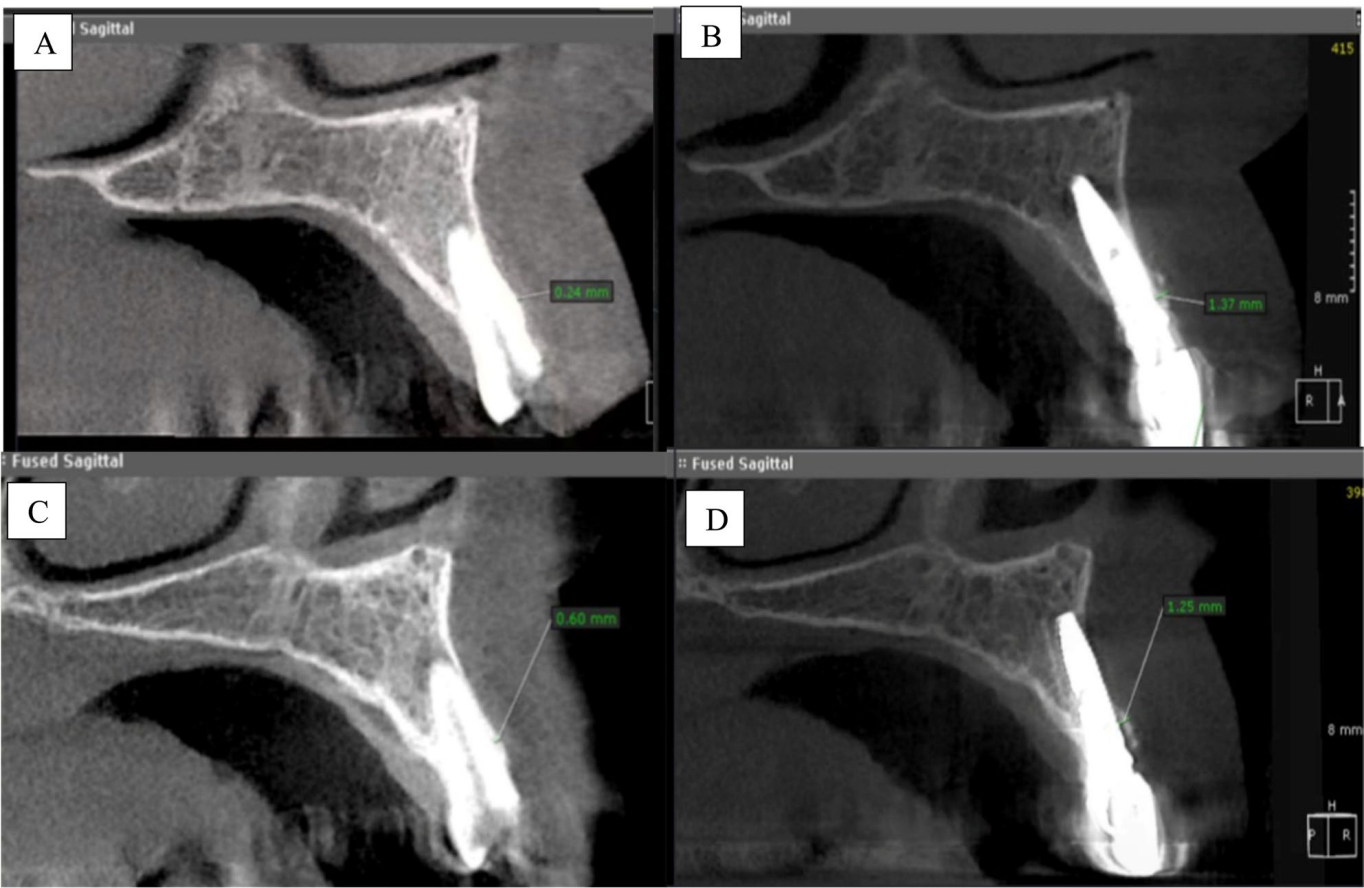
Radiographic superimposition to assess buccal cortex thickness (BCT). A a sagittal section revealing BCT before the surgical procedure. (B) a sagittal section of the superimposed radiograph revealing BCT changes after 6 months of using mVIP-CTG. (C) a sagittal section revealing BCT before the surgical procedure (D) a sagittal section of the superimposed radiograph revealing BCT changes after 6 months of using XCM
